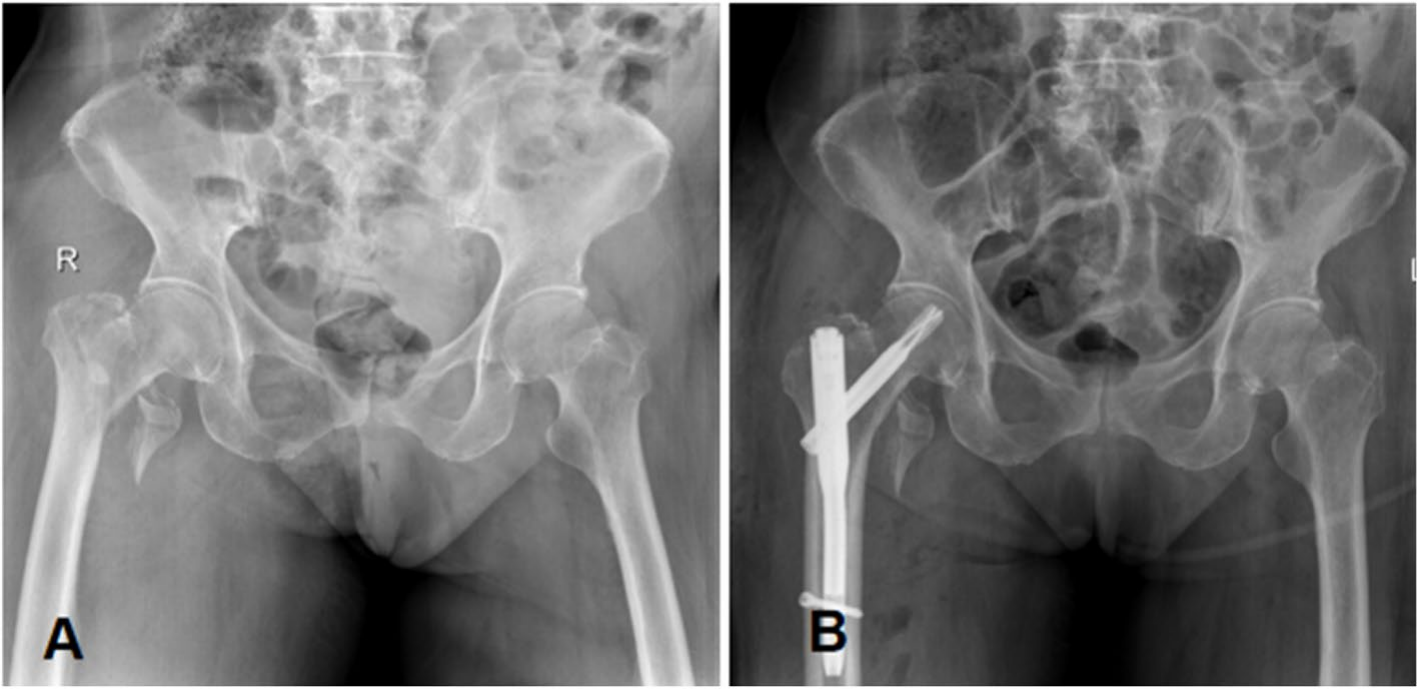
(A) pre-operative x-ray film of right femoral intertrochanteric fracture; (B) x-ray film after PFNA operation of right femoral intertrochanteric fracture
